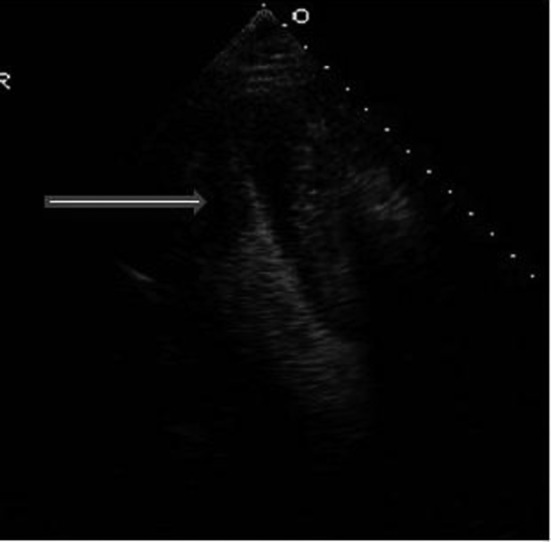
Transthoracic echocardiogram showing the apical hypertrophy of the left ventricle.
